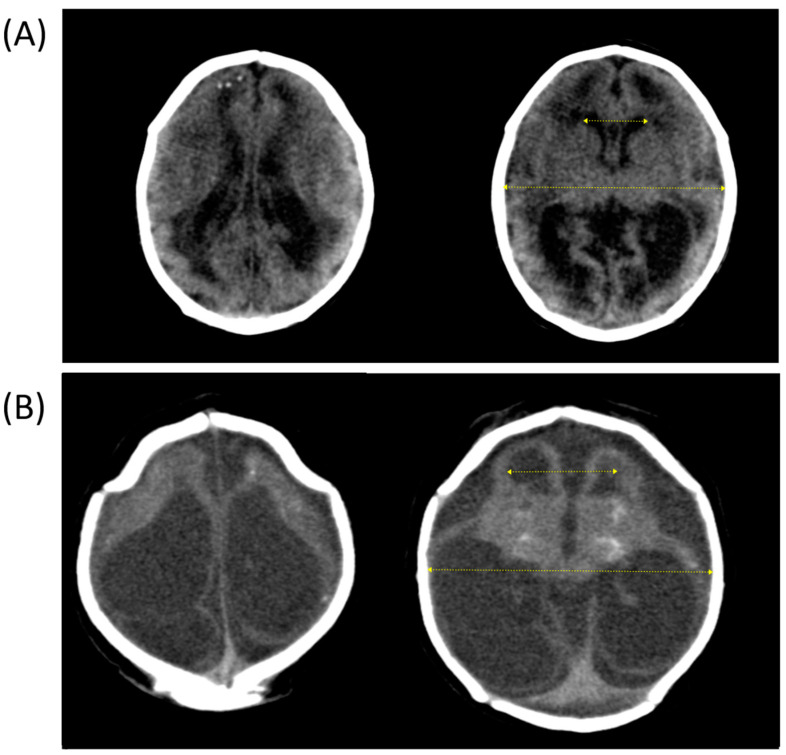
Head computed tomography showing the difference in the degree of brain damage between two children and their respective delays on Bayley Scales. (A) Evans’ index 0.32; delay of 23.9 months in cognitive, 22.9 months in receptive language, 23.0 months in expressive language, 22.0 months in fine motor, and 21.0 months in gross motor domains. (B) Evans’ index 0.41; delay of 41.8 months in cognitive, 39.9 months in receptive language, 41.8 months in expressive language, 41.8 months in fine motor, and 41.8 months in gross motor domains.
